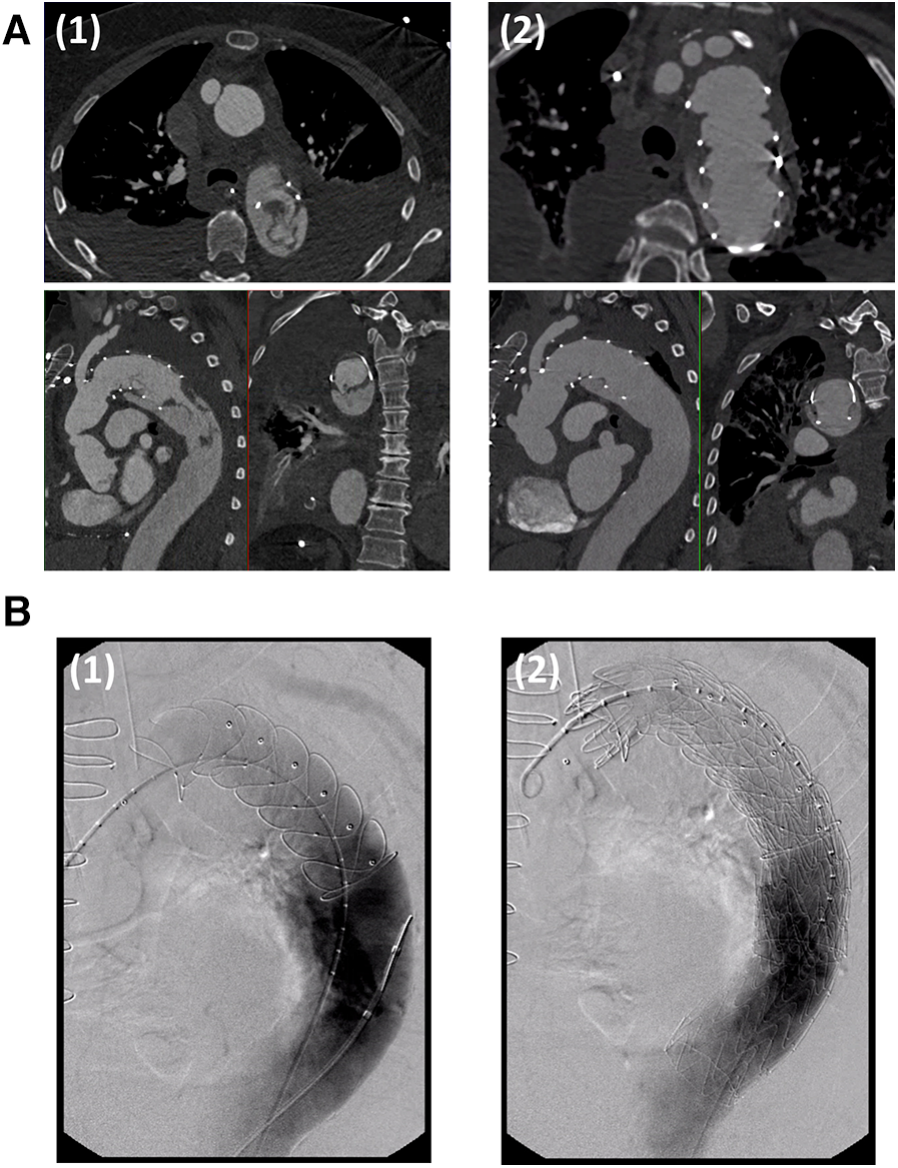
(A) CT-angiography of a patient with an intraluminal thrombus located at the distal end of the frozen elephant trunk stent graft diagnosed on the third postoperative day (1). Oral anticoagulation with a vitamin K inhibitor resulted in complete thrombus remission within 40 days (2). (B) TEVAR extension in a patient with mega-aortic syndrome and intraluminal thrombus formation after frozen elephant trunk implantation. The frozen elephant trunk stent graft is probed via a transfemoral access. Angiography showed an expected Type 1b endoleak (1). After TEVAR-extension, the endoleak was sealed and the intraluminal thrombus was excluded completely (2). Figures were adapted with permission from Martens et al.
